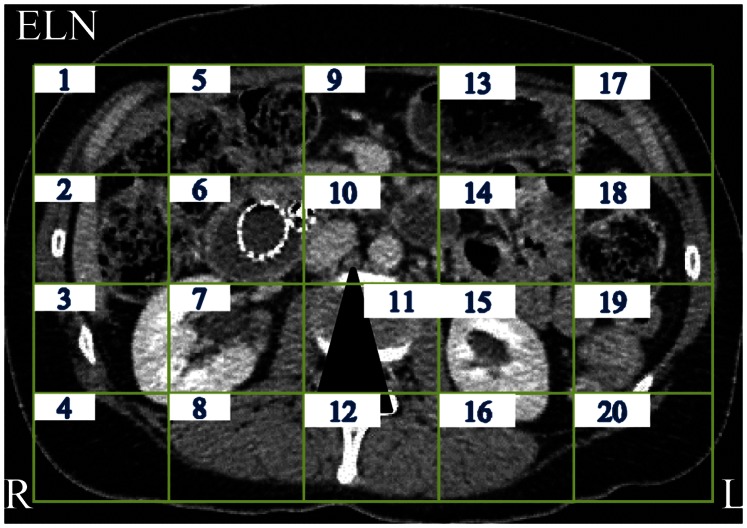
Cross-sectional abdominal CT-image with an ELN divided into 20 equal-sized areas of interest (AOIs). The enlarged lymph node in this image is pointed out by the black arrowhead and resides in Area 10, the area where most lymph nodes are located in all images across videos.
